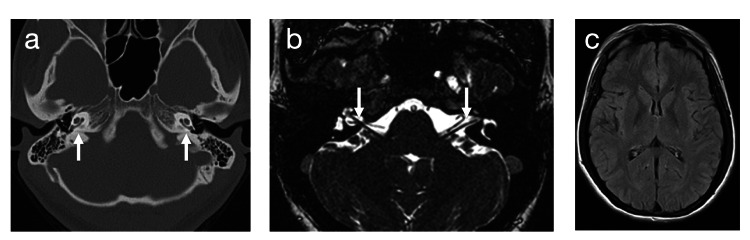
Computed tomography (CT) and magnetic resonance imaging (MRI). (a) Axial CT showing no abnormal morphology in both inner ears. Arrows indicate the cochleae on both sides. (b) Axial MRI showing intact bilateral cochlear nerves using fast imaging employing a steady-state acquisition technique. Arrows indicate the cochlear nerves within the internal auditory canals on both sides. (c) Axial T1-weighted MRI showing no evidence of encephalopathy.
